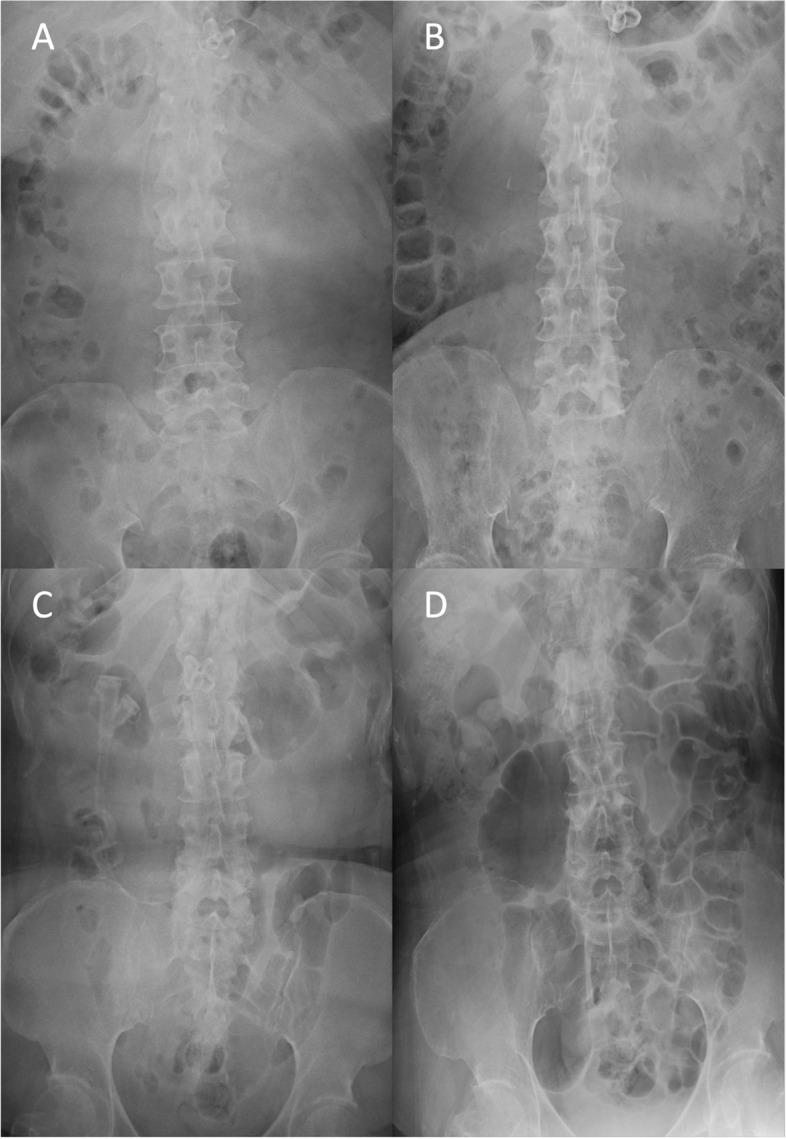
Abdominal X-ray in representative cases. A is an abdominal X-ray of Case 4 before central-part laryngectomy (CPL) showing the usual amount of intestinal gas; B is an abdominal X-ray of Case 4 20 months after CPL still showing the usual amount; C is an abdominal X-ray of Case 2 before CPL showing the usual amount of intestinal gas; D is an abdominal X-ray of Case 2 30 months after CPL with more than the usual amount of intestinal gas indicating evidence of aerophagia
